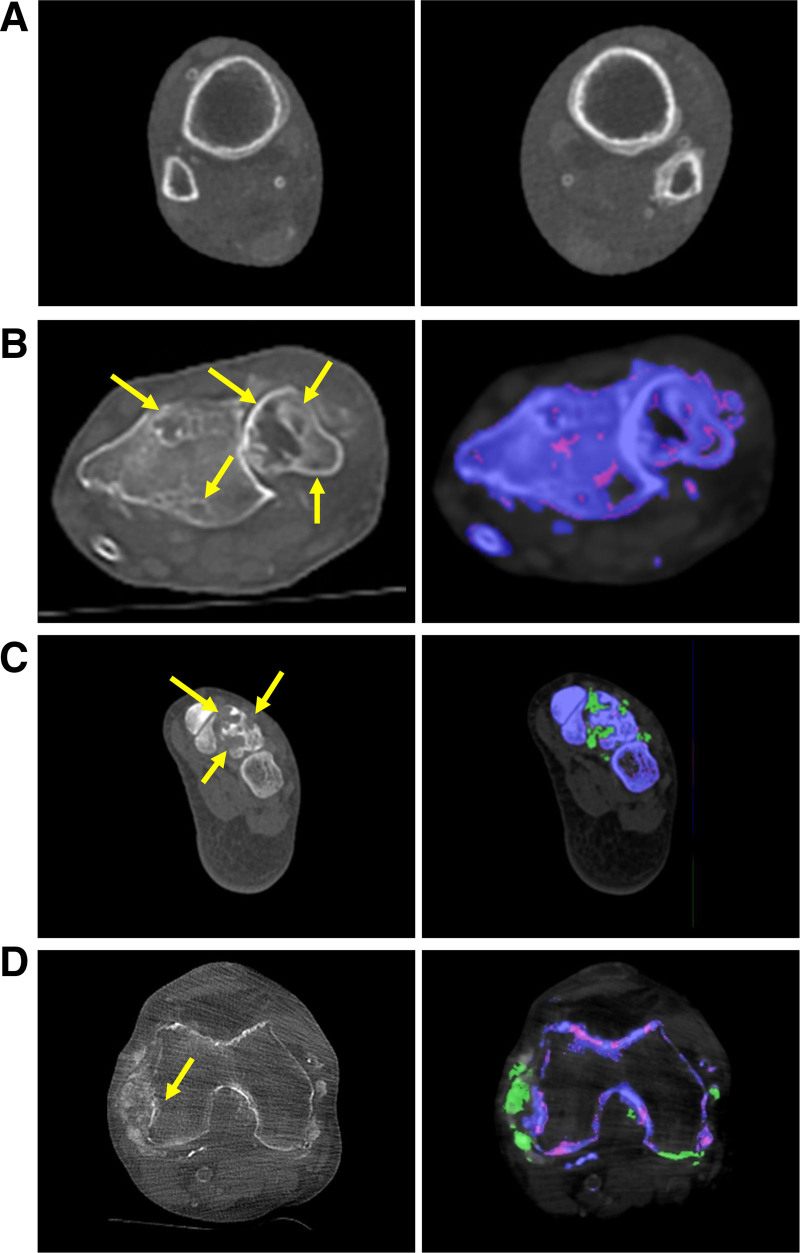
Bone-related DECT findings show osteopenia/osteomalacia (A) and multiple bone erosions (B–D) before pegloticase treatment. A, Axial CT image of the bilateral tibia and fibula before treatment demonstrates subperiosteal and intracortical resorption with diffuse osteopenia and periosteal reaction. B, Axial CT image of the wrist before treatment demonstrates multiple erosive-type lesions within the distal radius and ulna (yellow arrows). There is an amorphous mineralized matrix in the adjacent soft tissues. DECT images do not demonstrate that this mineralized matrix represents MSU deposits. C, Axial CT image of the bilateral feet demonstrates multiple erosions at the bases of the third and fourth metatarsals (arrows). DECT imaging reveals MSU deposits (green) adjacent to the erosions. D, Axial CT image of the knee demonstrates diffuse osteopenia. Amorphous mineralized matrix (white) is adjacent to the medial femoral condyle with adjacent erosion (yellow arrow). DECT imaging demonstrates both MSU deposits (green) and unknown mineralized material (whitish purple) adjacent to the bone erosion. DECT, dual-energy computed tomography; MSU, monosodium urate.
